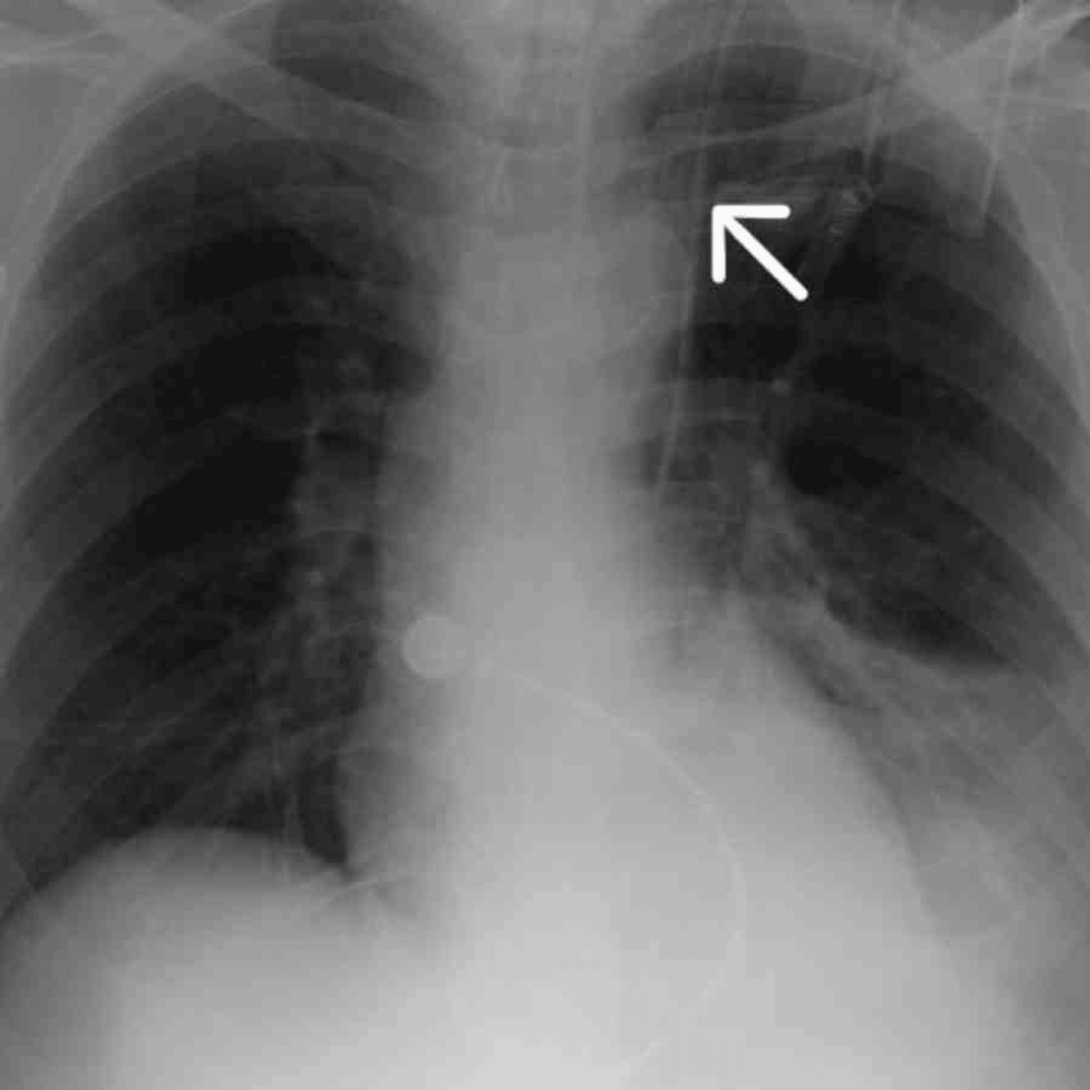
X-ray chest anteroposterior (AP) view The white arrow shows the catheter placement.
